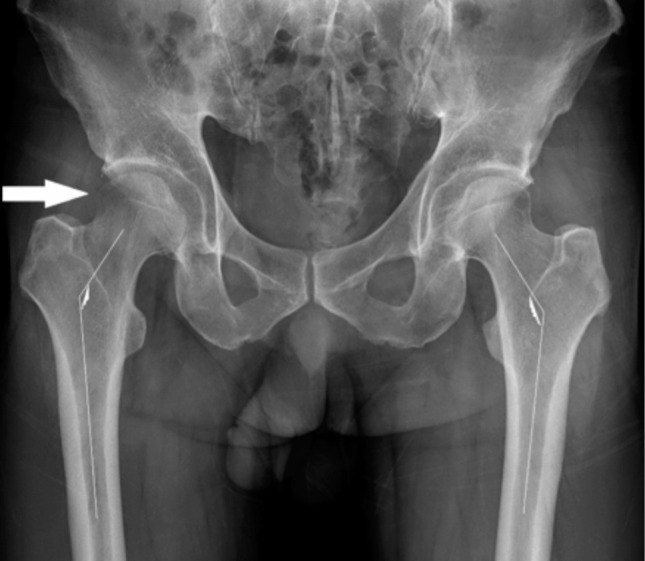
Anteroposterior pelvic radiograph showing osteopenic bone on right femoral head and neck (white arrow). Valgus neck-shaft angle indicated by the lines.
